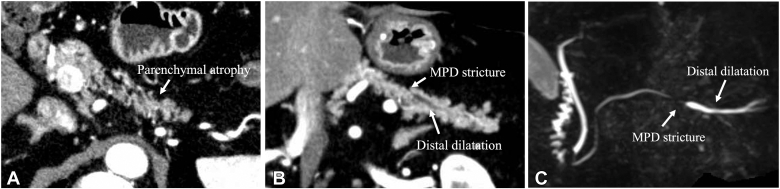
Contrast-enhanced CT and MRCP findings. A and B, Axial and coronal CT images showing the MPD stricture and parenchymal atrophy in the pancreatic tail. C, MRCP revealing the MPD stricture and distal dilatation. *CT*, Computed tomography; *MPD*, main pancreatic duct; *MRCP,* magnetic resonance cholangiopancreatography.
